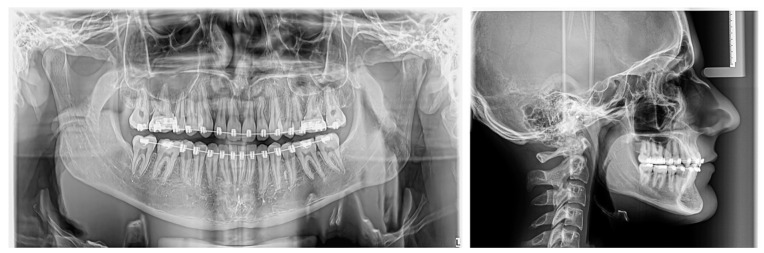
Panoramic radiograph and lateral cephalometric radiograph before debonding.
